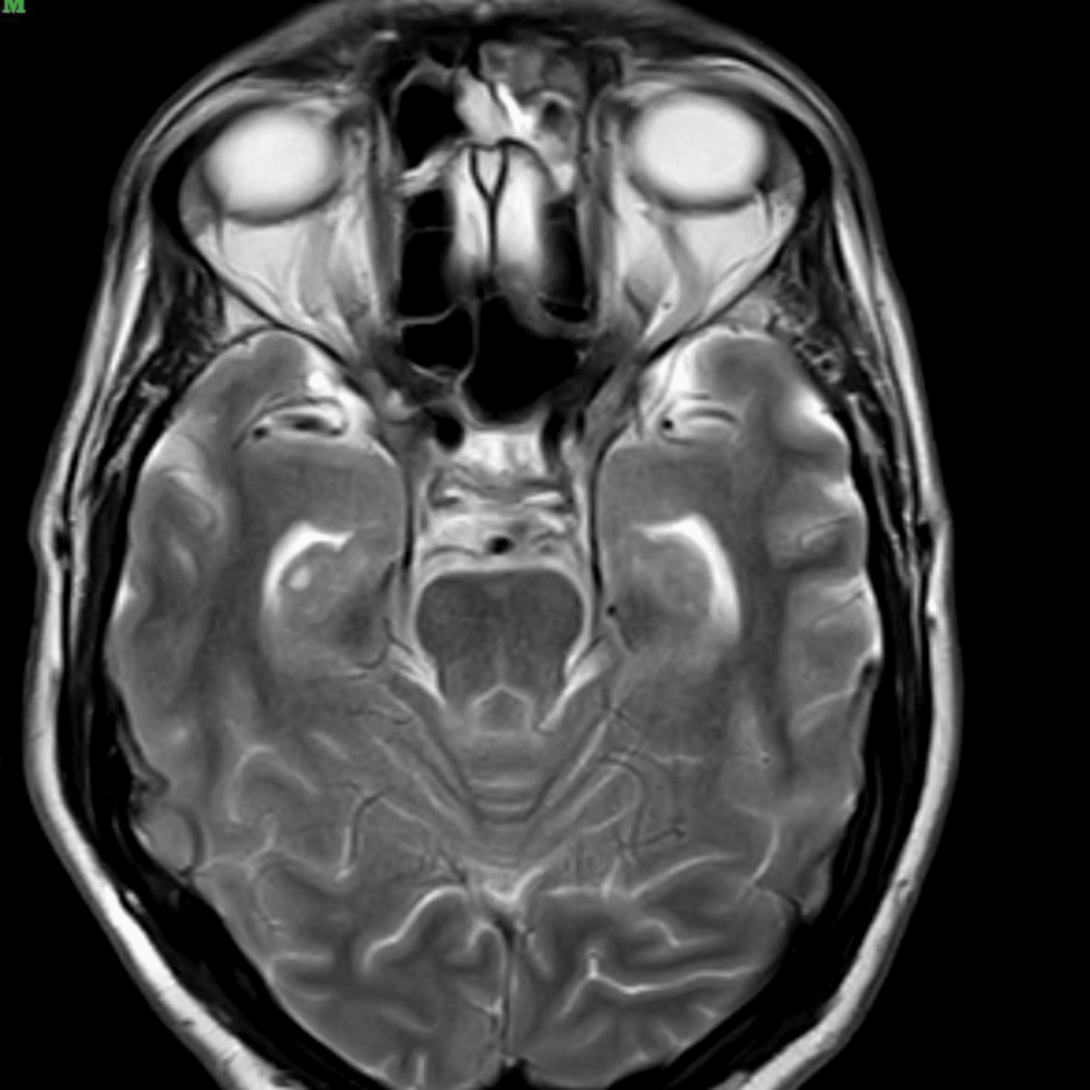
Axial T2-weighted MRI demonstrates hyperintense signal changes in the bilateral mesial temporal lobes and basal ganglia, with prominent CSF spaces
